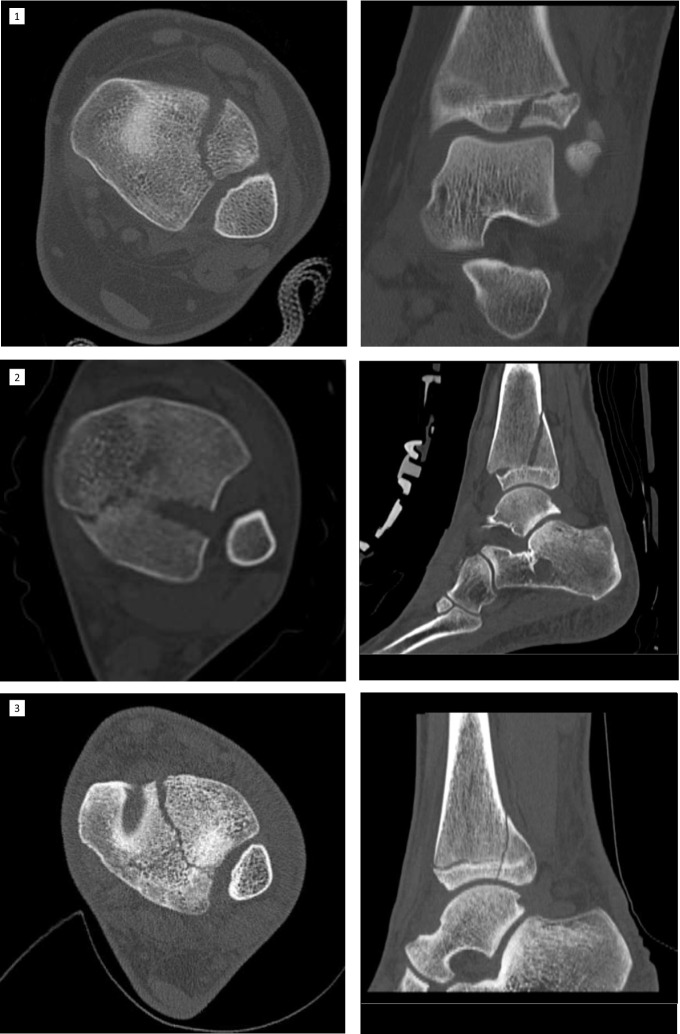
Preoperative CT images. The fracture line can be distinguished exactly by CT or MRI. Here are shown CT images of Twoplane (1), Triplane I (2) and Triplane II (3) fractures
